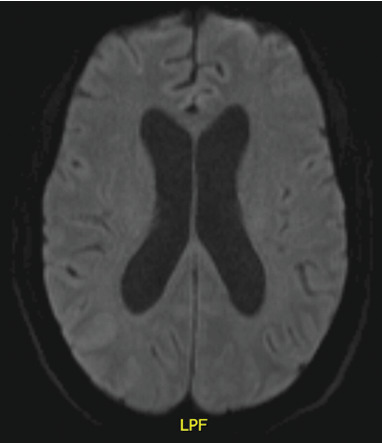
MRI of the brain (DWI-DTI) showing no evidence of neoplastic involvement. Axial diffusion–weighted and diffusion tensor imaging (DWI-DTI) sequences demonstrate no definite hydrocephalus or ventricular obstruction. There is no radiologic evidence of cranial neoplastic disease or leptomeningeal enhancement, despite positive cerebrospinal fluid cytology for malignant cells (original image, Department of Medical Imaging, Western University).
